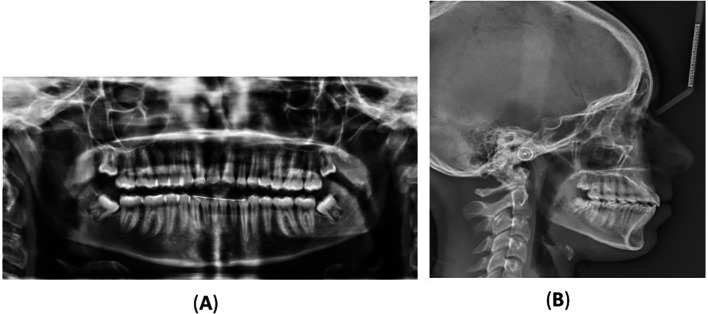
Final radiographs: (A) orthopantomography and (B) lateral cephalometric.
